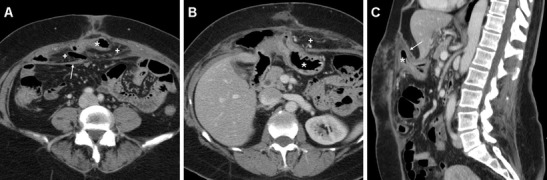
Failed repair of large ventral incisional hernia in a 50-year-old obese female. Four days after surgery, urgent CT was obtained because distended, tender abdomen revealed detachment of the PM (thin arrows) from the anterior abdominal wall, and recurrent ventral herniation of intra-abdominal fat (+) and of a portion of the gastric antrum (*)
